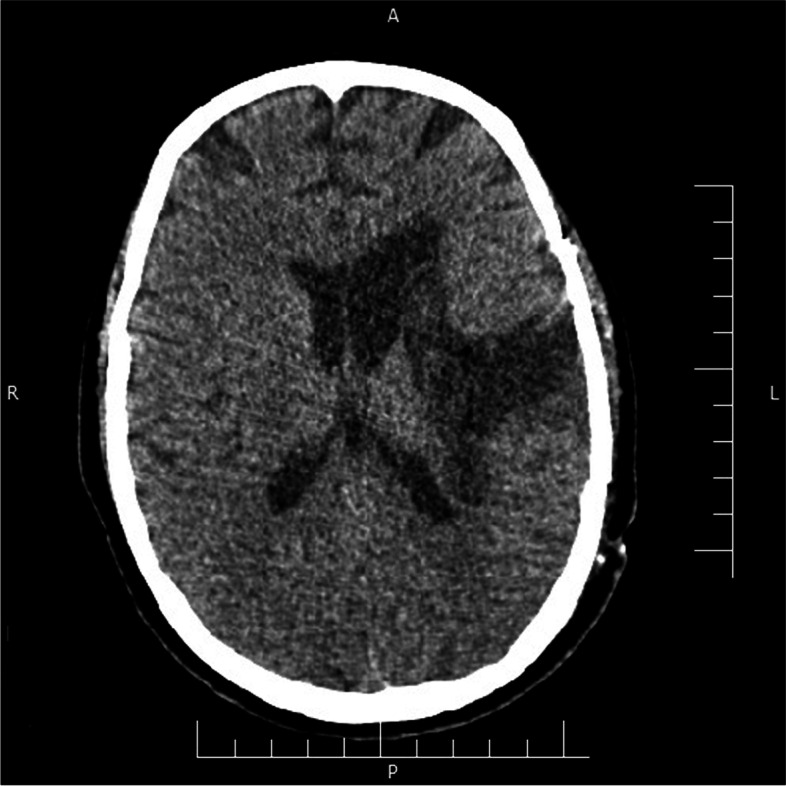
Brain CT scan showed no signs in favor of rebleeding at the one-week follow-up
